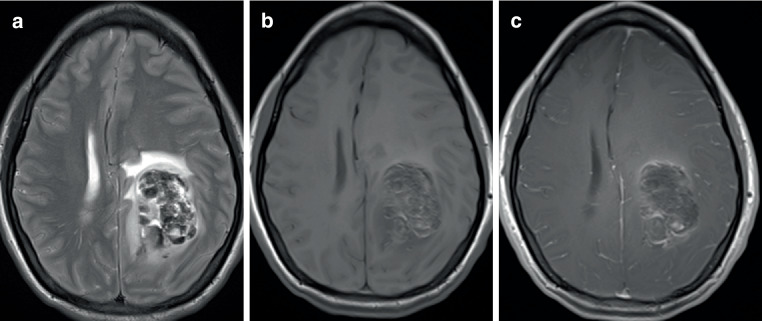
a–c Diffuse hemispheric glioma with mutation of the histone 3-3A gene at position 34 (H3G34-mutant) (female, age: 33 years) with involvement of the left hemisphere parietal showing heterogeneous signal intensities in T2-weighted and T1-weighted sequences (a, b) with hemorrhages and only faint contrast enhancement on T1-weighted image after i.v. application of contrast agent (c). a T2-weighted image, b T1-weighted image, c T1-weighted image after i.v. application of contrast agent. Molecular analysis revealed a H3G34-mutation of the H3.3 gene with amino acid substitution at position 34. This mutation is pathognomonic for a diffuse hemispheric glioma, H3G34-mutant
